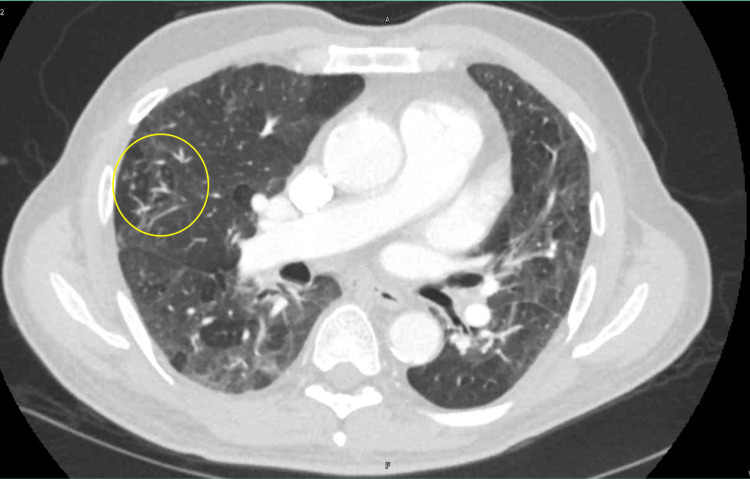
The CT thorax with contrast from 2023 highlights tree-in-bud nodularity in the right middle lobe (circle).
